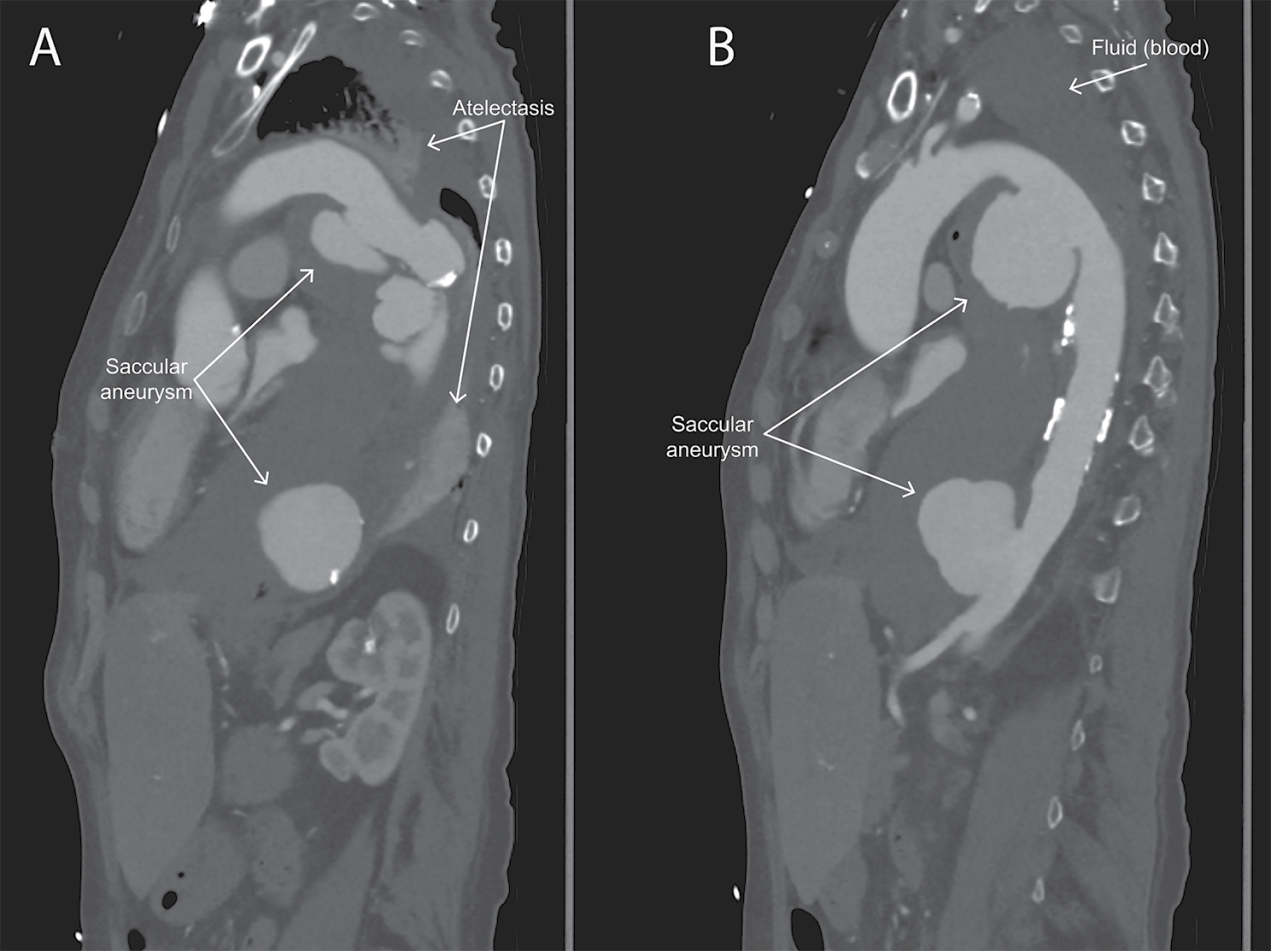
Two sagittal plane CT-scan images of the ascending and descending aorta with the two saccular aneurysms in the proximal (A) and distal (B) part of the thoracic descending aorta. Also visible is the fluid (blood) that has filled up the whole left hemithorax and replaced the left lung (which is atelectatic)
